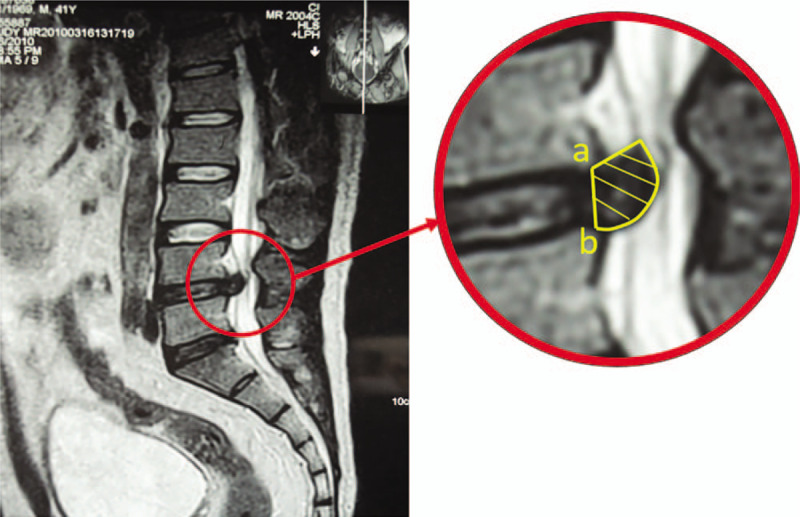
Representative MR image to calculate the resorption rate. MR = magnetic resonance.
